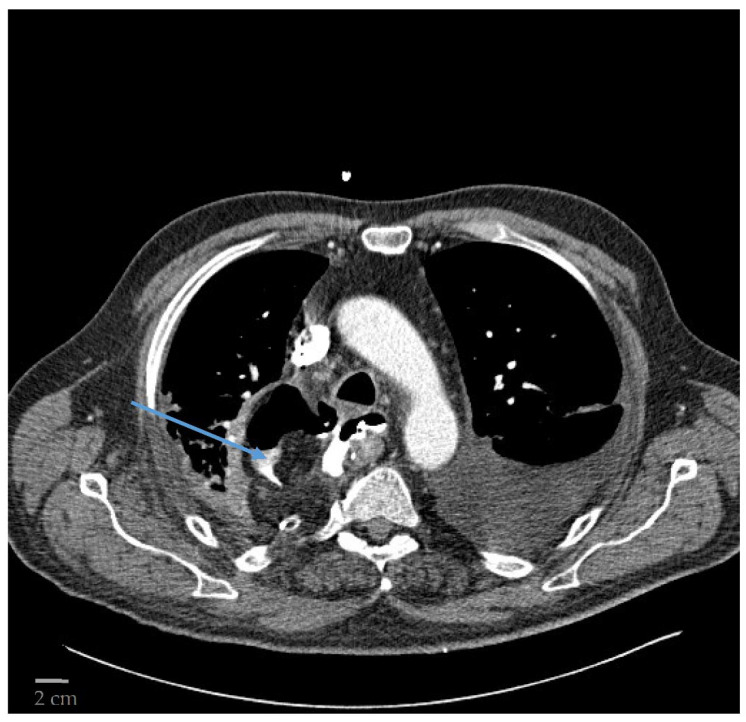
CT displaying an AL with extravasation of contrast agent and free air in the mediastinum indicated by the blue arrow.
